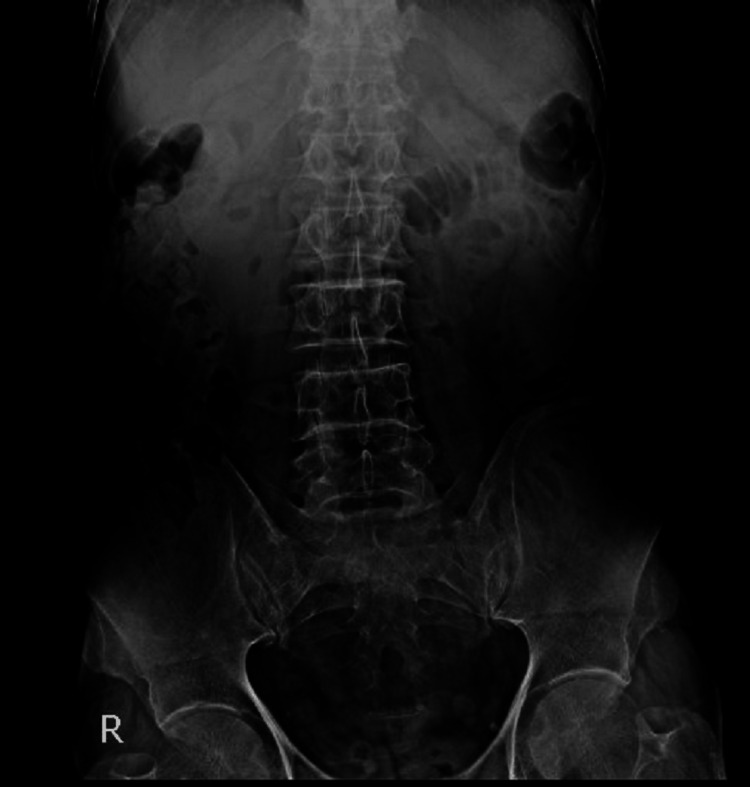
Upright abdominal X-ray showing mildly dilated small bowel loops
